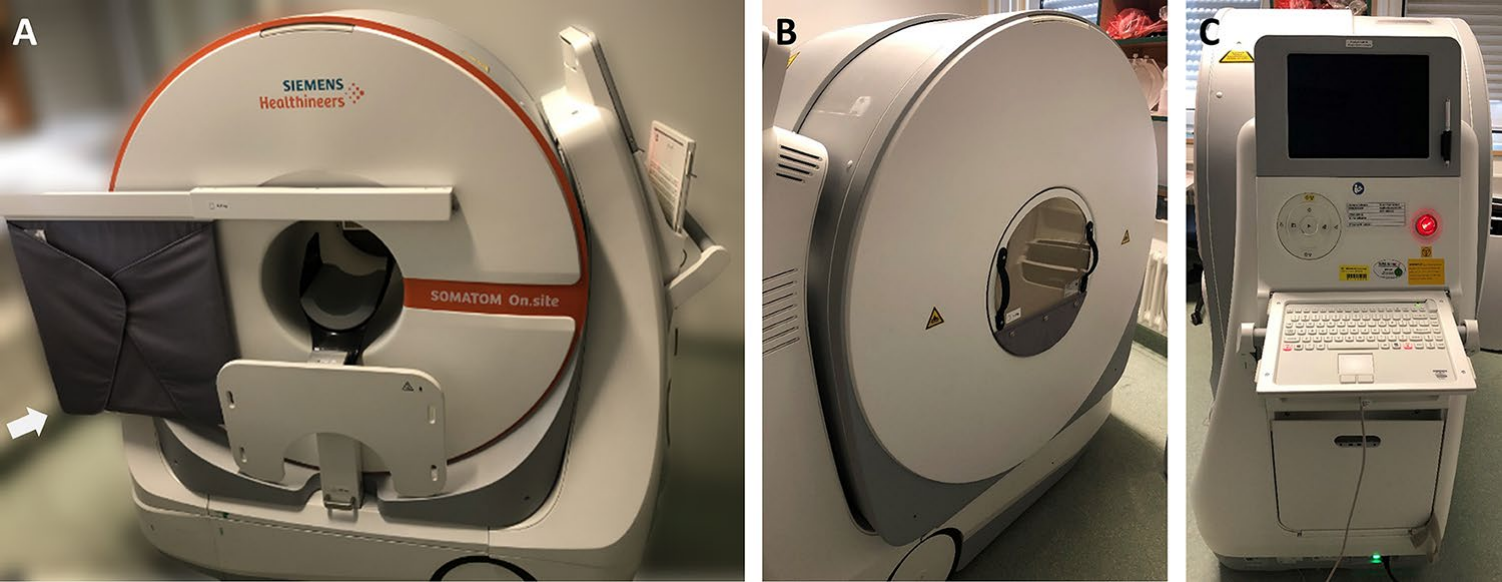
Images of the Siemens SOMATOM On.site taken at the storage location in the NICU with frontal (A), oblique (B) and lateral (C) views. The CT scanner weighs 890 kg but can be moved and operated by one person. It is equipped with a keyboard and touch screen for operating the unit and viewing reconstructed images of the scan at the bedside (C). A lead apron is fixed to the scanner (A, block arrow), which can be moved for radiation protection of the adjacent patient.
